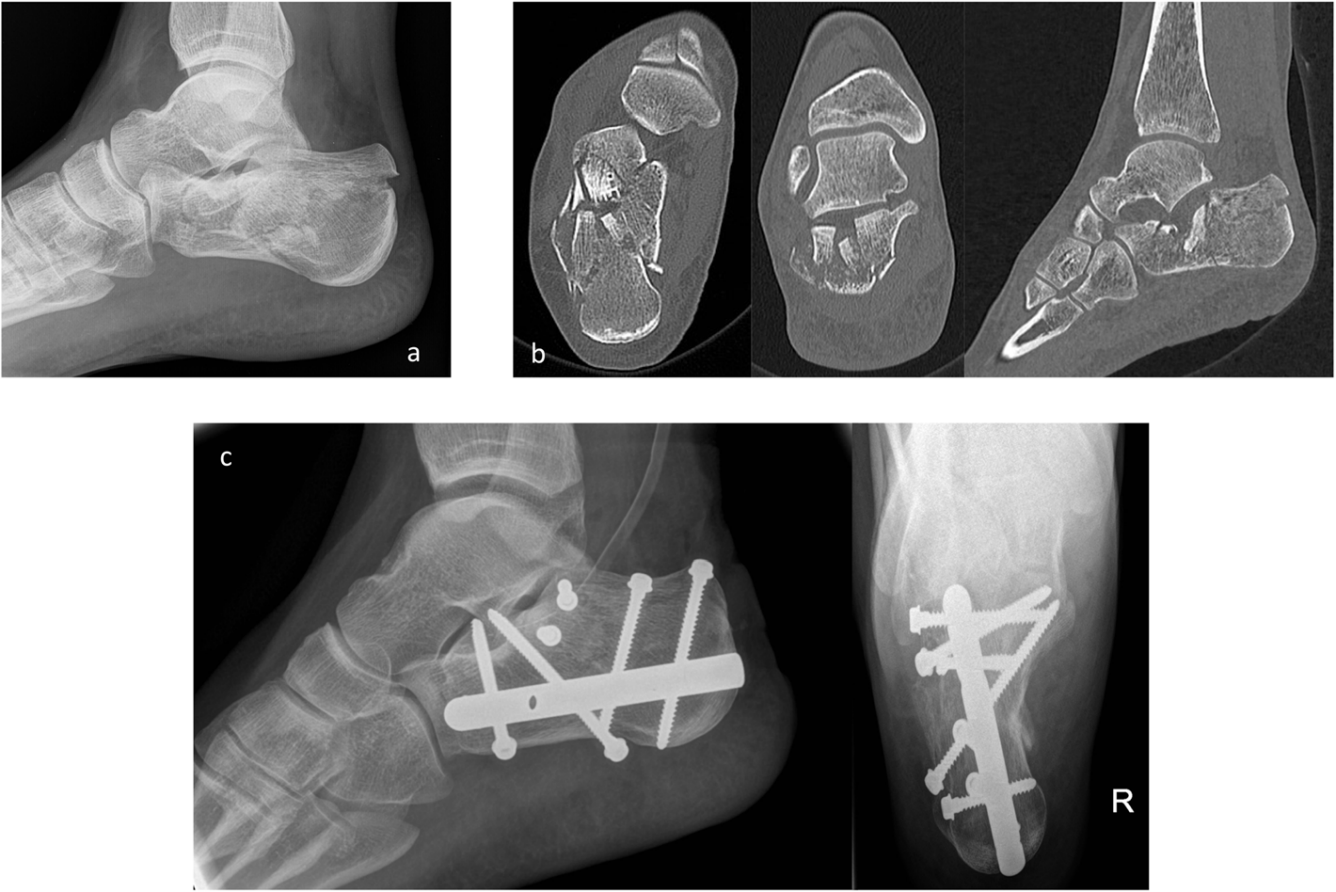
Calcaneal fracture, osteosynthesis with C-nail. a Preoperative x-ray. b Preoperative CT scan. c Osteosynthesis with C-nail, postoperative x-ray
